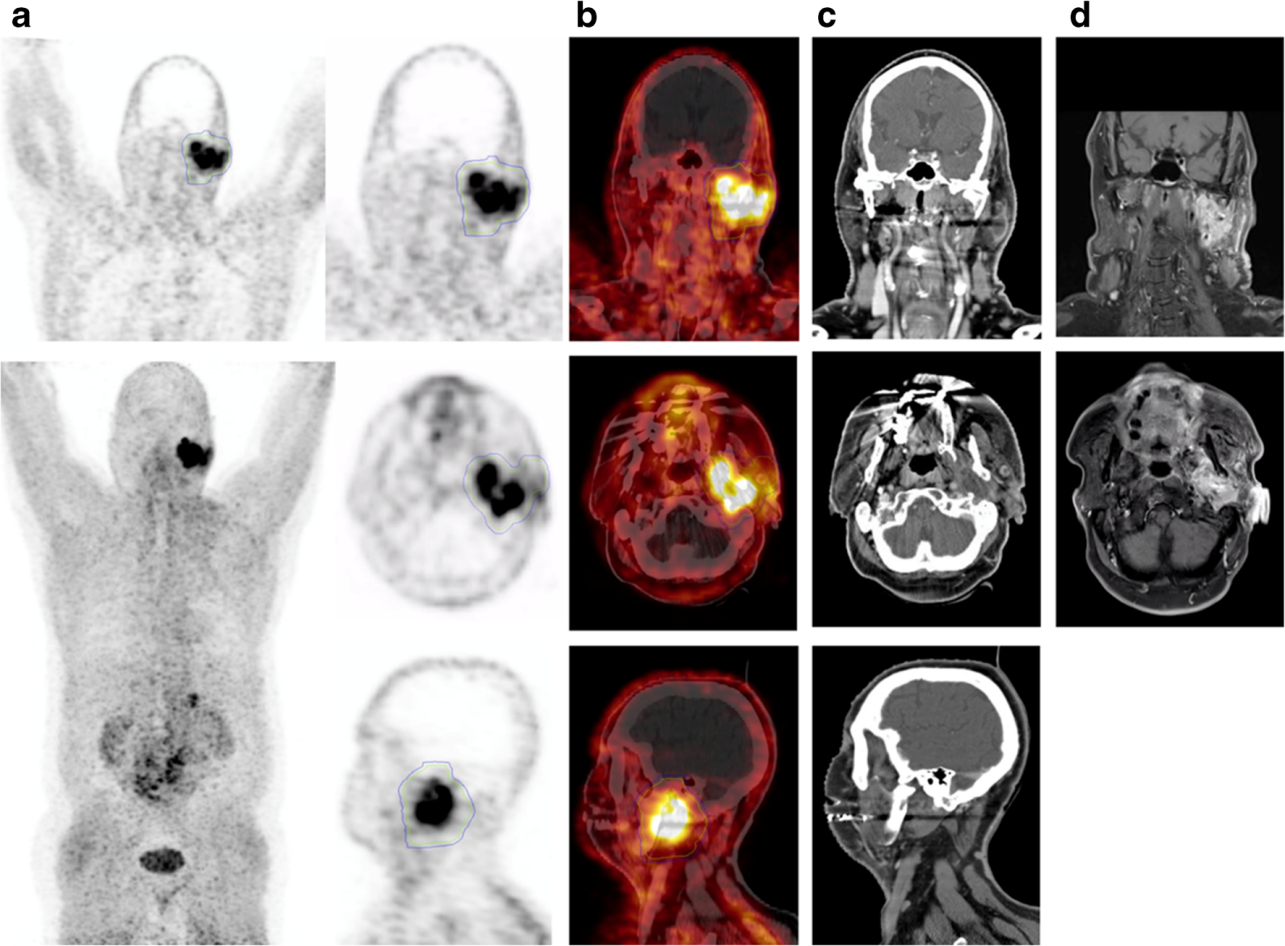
Example of a 64-year-old male patient with mucoepidermoid carcinoma of the left parotid gland receiving FAPI-PET CT and radiation treatment. a, b MIP and CT-fused FAPI-PET images showing precise tracer uptake by the parotid carcinoma and very low background noise. c, d Conventional CE-CT and CE-MR imaging showing diffuse tumour infiltration making differentiation between tumour and healthy tissue enormously difficult and subjective. Abbreviations: CE, contrast enhanced; FAPI-PET, ^68^Ga-fibroblast activation protein inhibitor PET-CT
